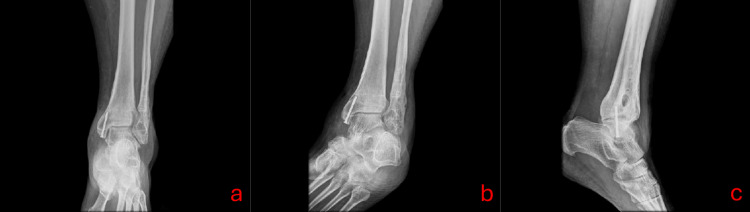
Anteroposterior (a), oblique (b), and lateral (c) radiographs of the left ankle. Bony alterations are observed in the distal tibiofibular syndesmosis region and the areas corresponding to the previous osteosynthesis material. Radiolucent tubular images with well-defined edges are identified, consistent with the previous TightRope pathway. Peripheral sclerosis is noted at the margins of the tunnels, without evidence of active lysis or osteolytic reaction.
